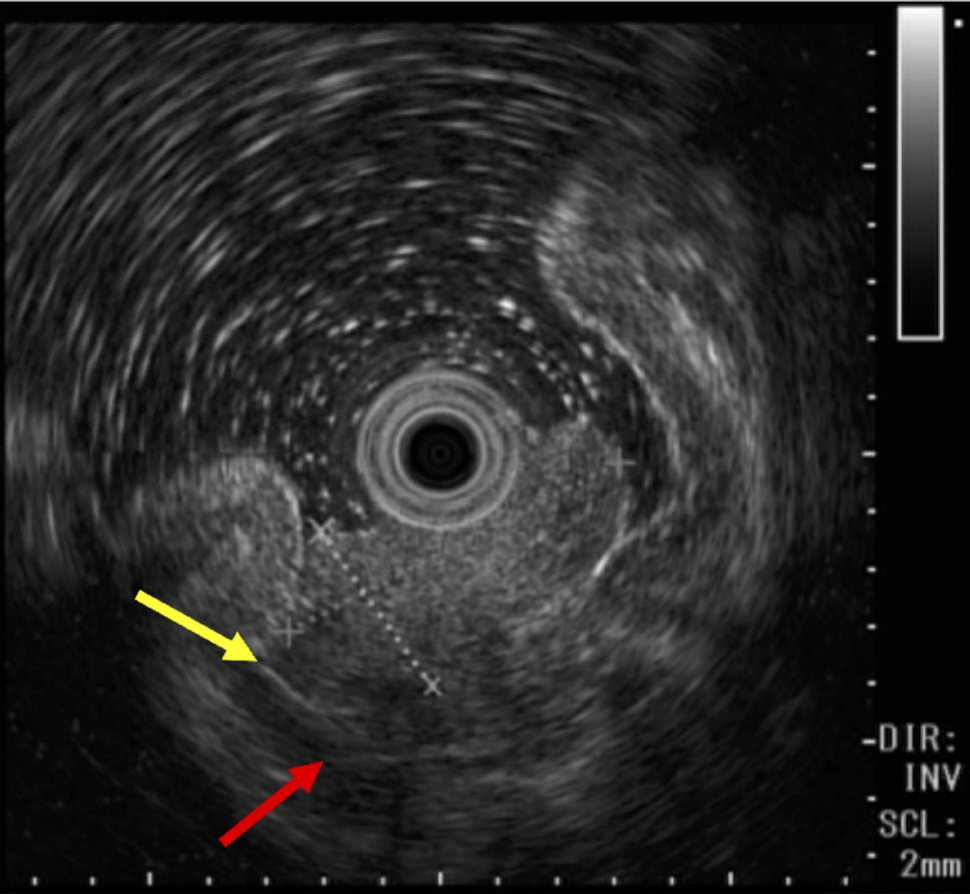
Endoscopic ultrasonography (EUS) images of the lesion. The hyperechoic area indicated by the yellow mark corresponds to the submucosal layer, while the hypoechoic area indicated by the red mark corresponds to the muscularis propria. EUS demonstrated a mixed-echo lesion originating from the proper mucosal or submucosal layer, raising concern for possible submucosal invasion. Although submucosal invasion by the tumor was suspected due to partial obscuration of the submucosal layer, the possibility of muscularis propria invasion was considered unlikely in this case, as there was no evidence of wall thickening in the muscular layer
